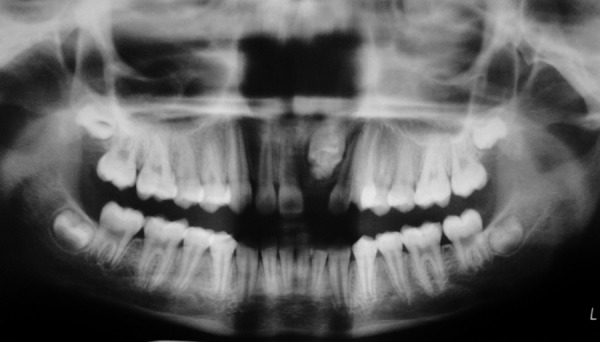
Preoperative panoramic radiograph showing multilocular radiopacity
